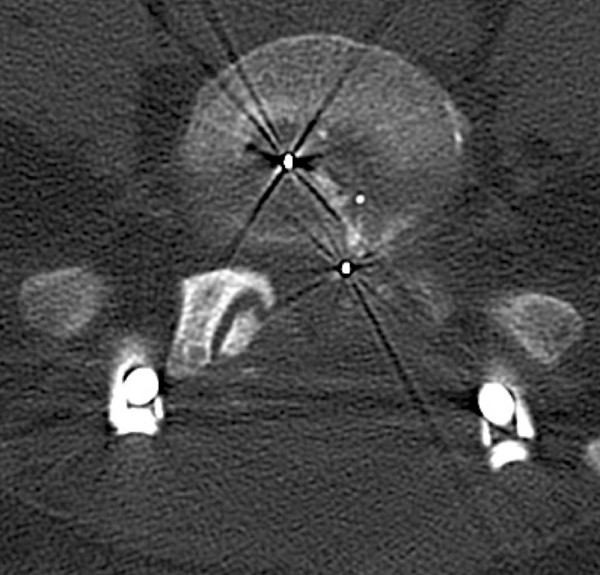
Computed tomographic scan taken at first follow-up examination.
